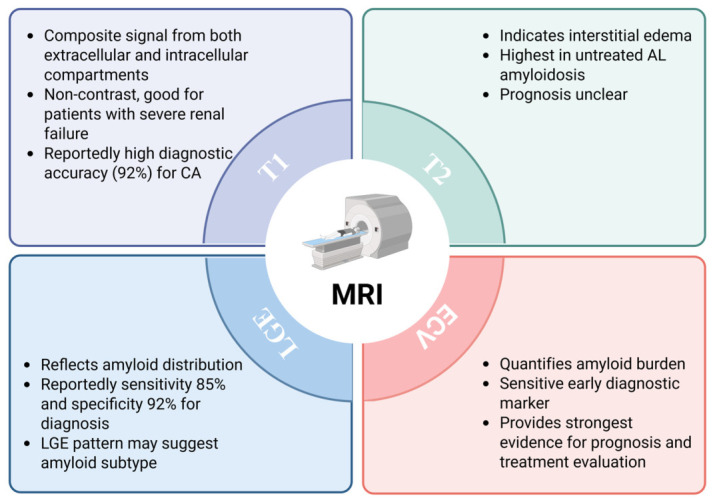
Application of magnetic resonance imaging and key parameters in AL amyloidosis. CA, cardiac amyloidosis; AL, light chain; LGE, late gadolinium enhancement. Created in BioRender. Qiu, M. (2026). https://BioRender.com/5nrmmye (accessed on 26 May 2026).
